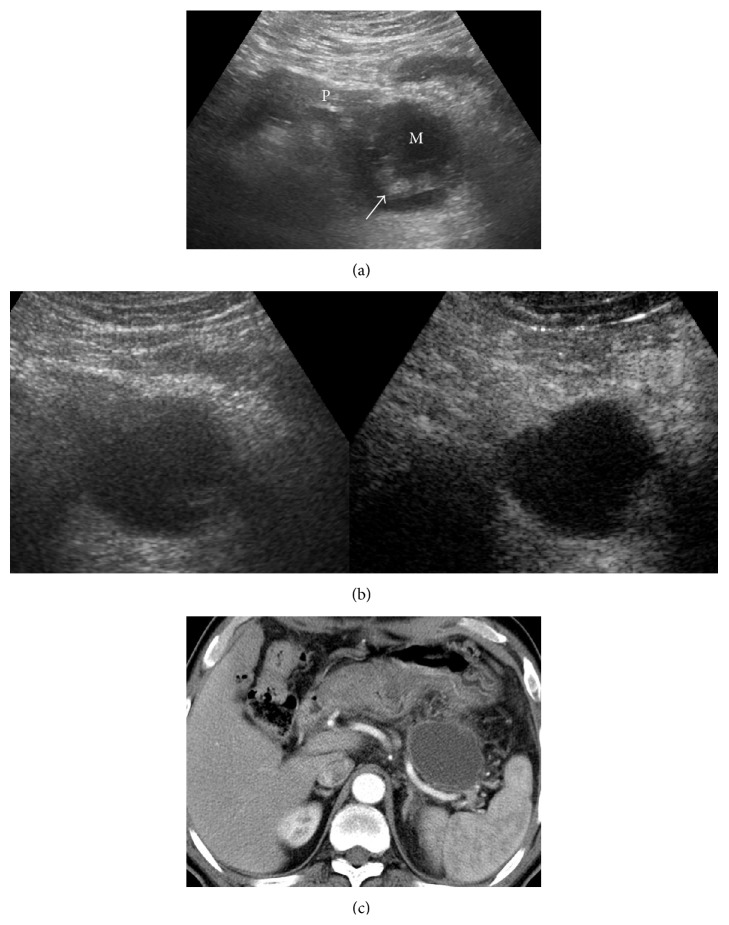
Pancreatic lesion was found by follow-up after acute pancreatitis. The case was diagnosed as a pseudocyst by surgical pathology. (a) US indicated cystic lesion in the tail of the pancreas (M: mass; P: pancreas), which contained low-echo solid components (arrow). The case was diagnosed as type IV by US. (b) There was no enhancement inside the cystic lesion in CEUS (the right picture). The case was diagnosed as type I by CEUS. (c) Enhanced CT indicated no enhancement in the cystic lesion. The case was diagnosed as type I.
